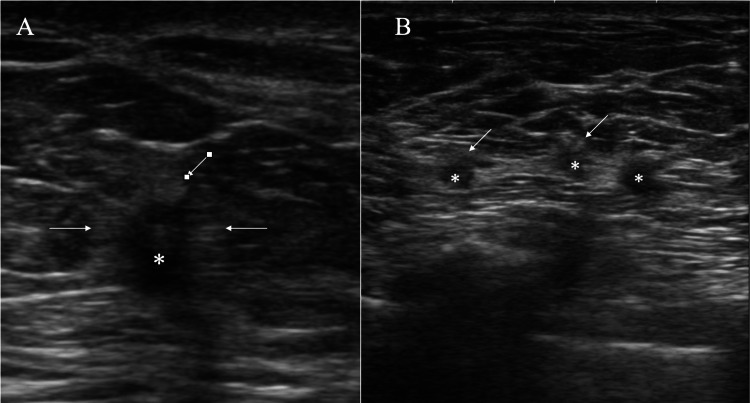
Ultrasound findings of the axillary lesions. A. Ultrasound showed the largest lesion (asterisk), less than 1cm in size, with predominant internal low echoes and haloes (arrows) surrounding the mass; B. Ultrasound showed multiple small lesions(asterisks) with haloes (arrows) lining in line.
